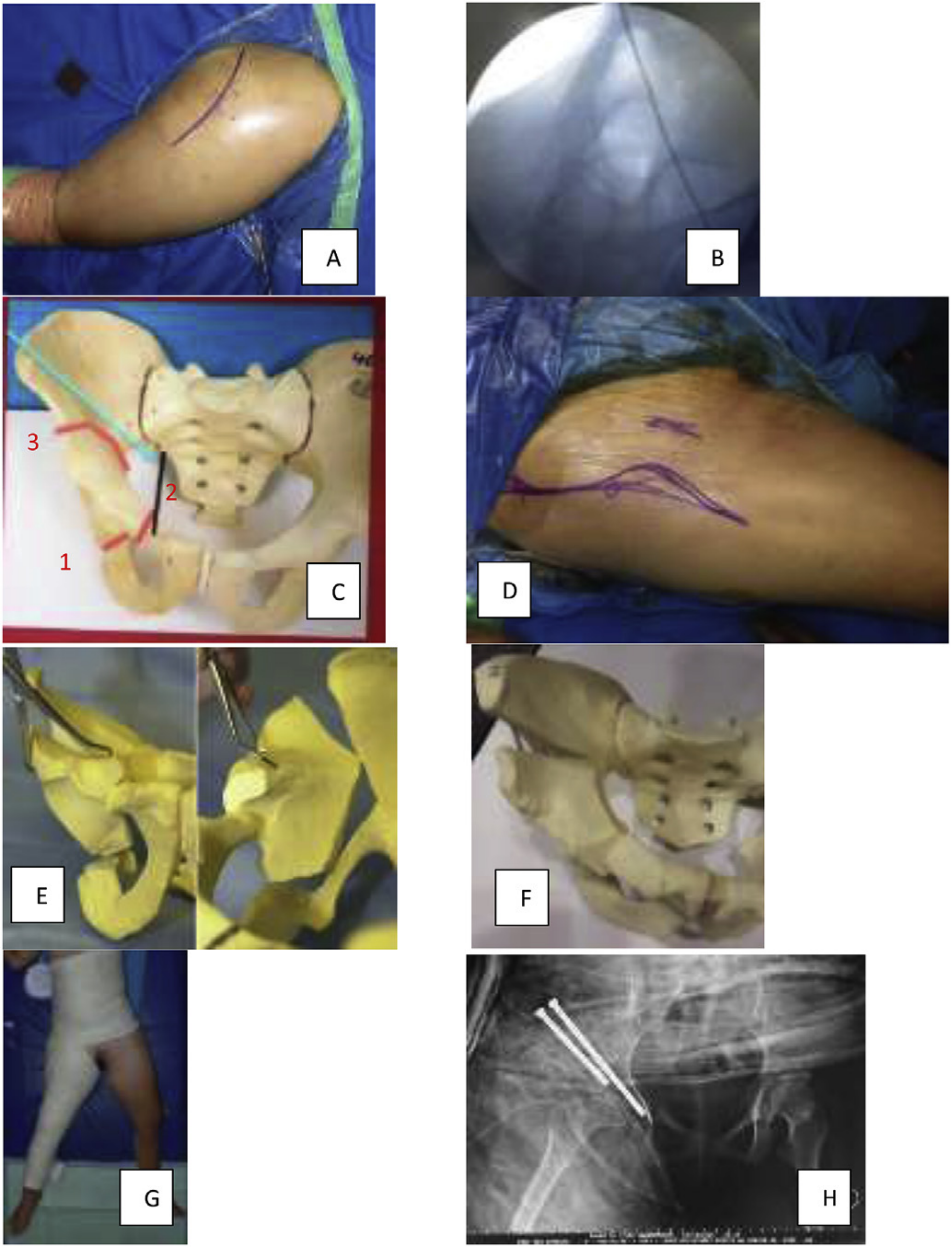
Surgical technique of modified PAO. A, patient was positioned in lateral decubitus; B, hip was reduced and confirmed using image intensifier (II); C1-3, osteotomy of ischium was performed first then followed with superior ramus pubis and acetabulum using anterior approach; D, patient then positioned supine and anterior approach were performed to proceed with C2-3; E, derotation was performed; F, fixation using cannulated screw and bone graft application; G, post-operative hemi spica cast was applied and; H, post-operative xray showing increased well contained hip.
